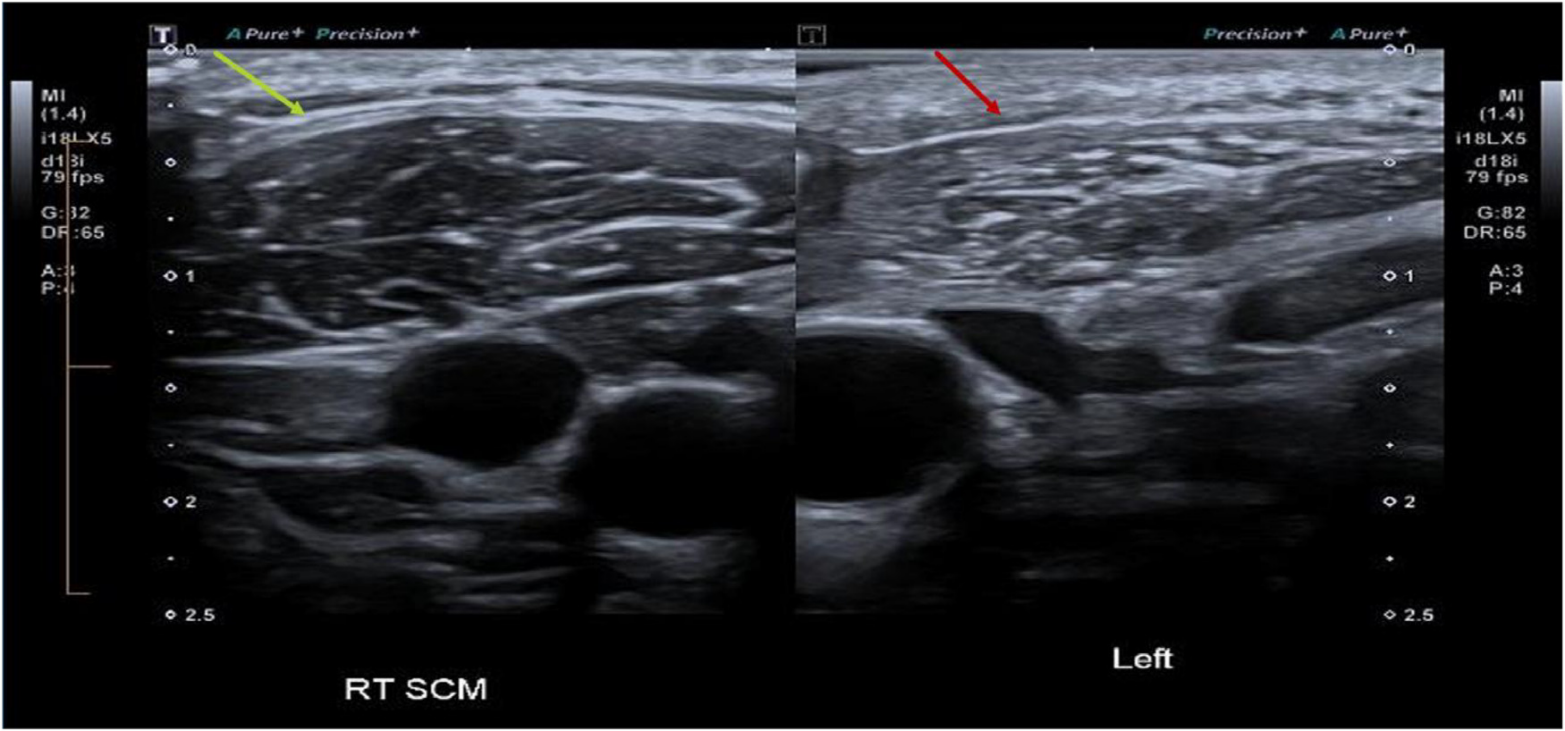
Ultrasound images demonstrating marked reduction in muscle volume and fatty infiltration of the left sternocleidomastoid muscle (SCM), red arrow, in comparison to the right SCM muscle, yellow arrow.
